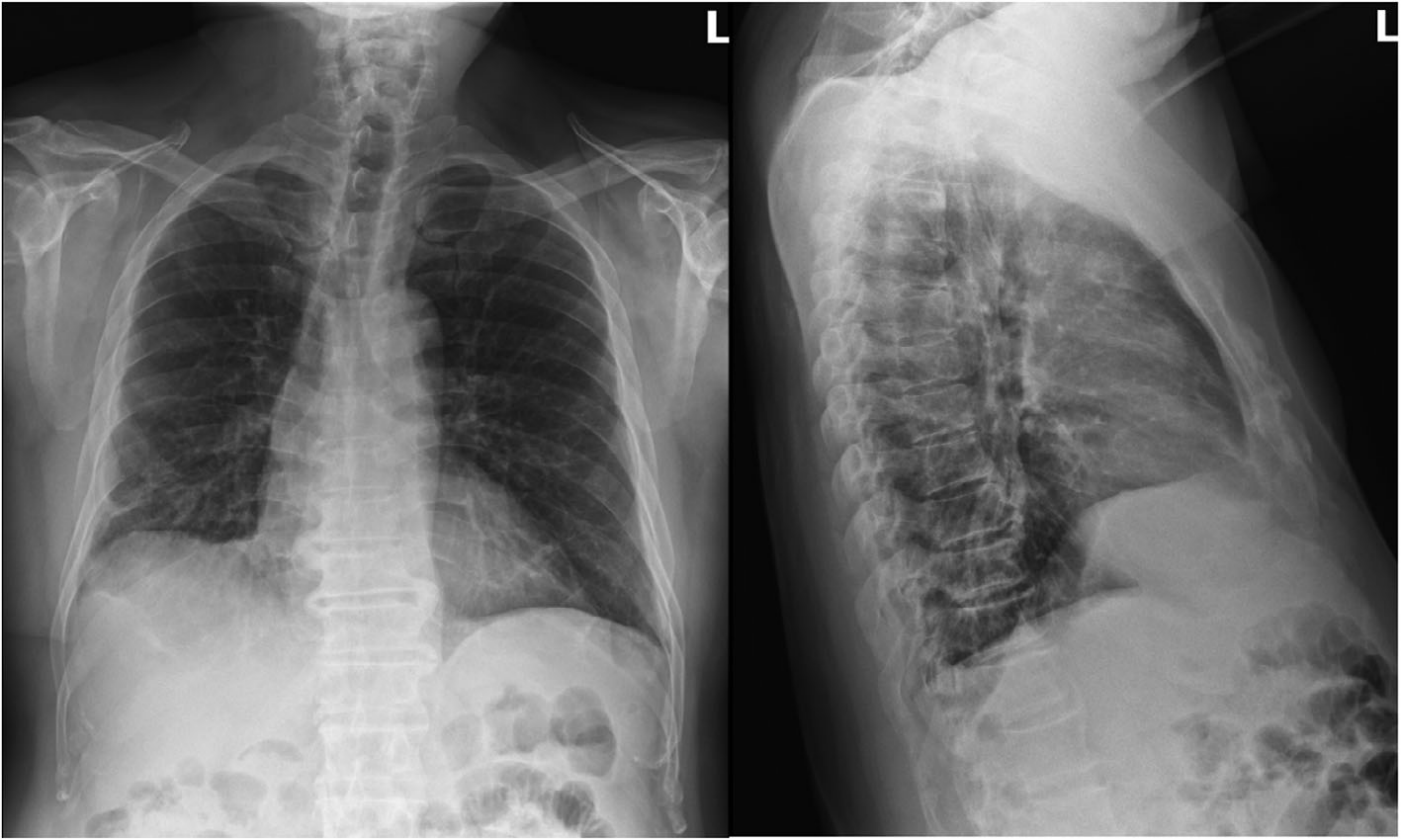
Chest X‐ray in June 2021: Improved aeration of the lungs with decreasing peripheral based opacities, compared to CT chest done in April 2021, most notably in the right lung. No new focal consolidation, effusion, or pneumothorax.
